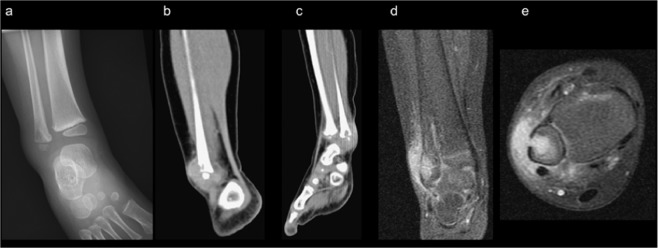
Lytic lesion in the metaphysis of the right distal fibula alongside cortical destruction and a partial osteosclerosing rim. (a) Osteolytic bone lesion in the metaphysis abutting to the physis surface of the right distal fibula and focal lucent bone density of the epiphysis of the distal fibula alongside an adjacent swelling inflammatory mass with peripheral enhancement, measuring approximately 2.7 × 2.6 × 1.6 cm on a computed tomography scan (b,c). Bone marrow edema in the right distal fibula, surrounding soft tissue swelling, and intraosseous fluid collection with a drainage sinus tract to the extraosseous region, measuring approximately 1.7 × 1.5 × 1.2 cm in magnetic resonance imaging (d,e).
